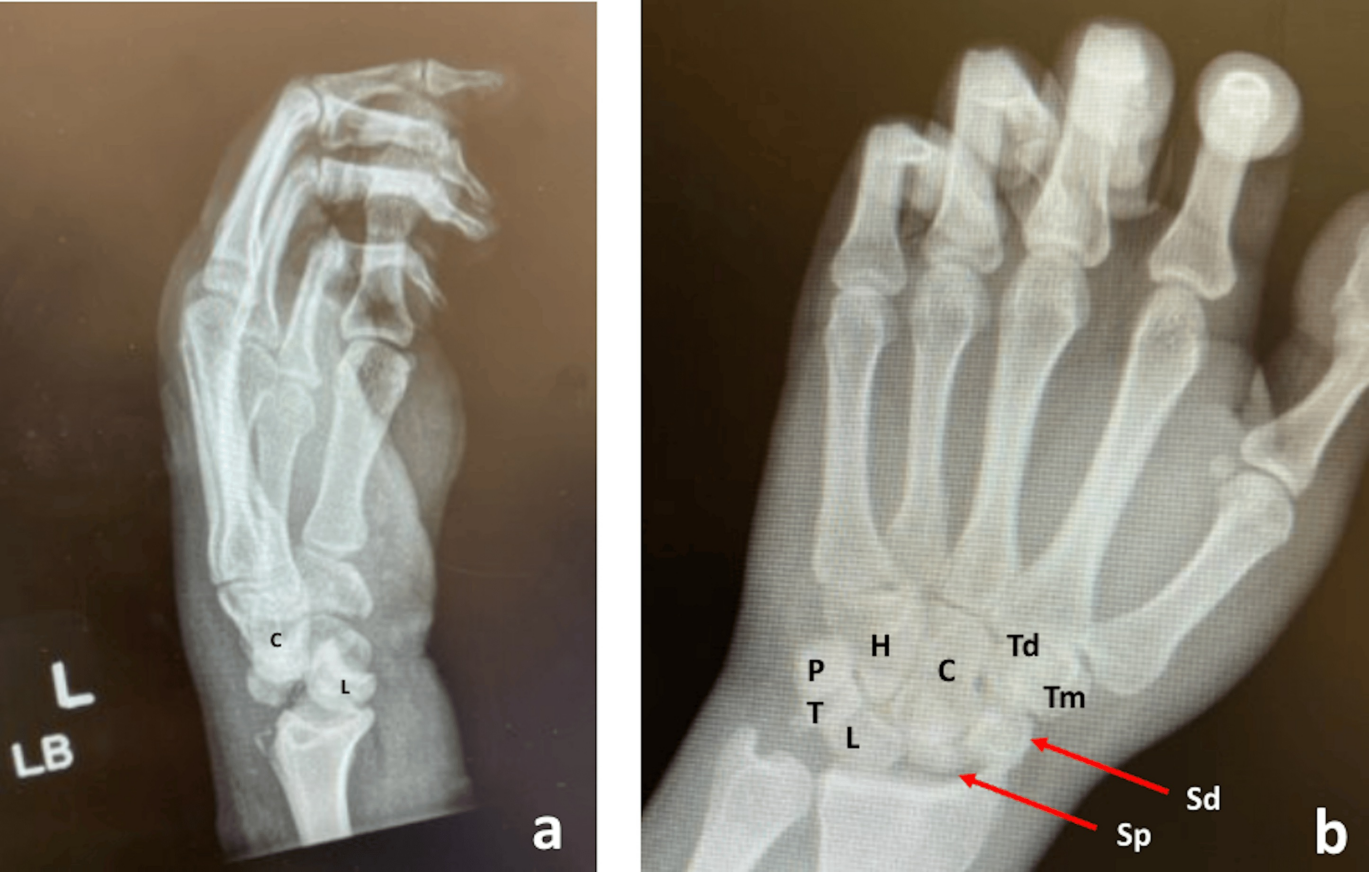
Lateral (a) and posteroanterior (b) view of radiographs showing lunate dislocation and scaphoid fragments. Sd, distal pole of fractured scaphoid; Sp, proximal pole of fractured scaphoid; LB, lateral beam; L, lunate; T, triquetrum; P, pisiform; H, hamate; C, capitate; Td, trapezoid; Tm, trapezium.
